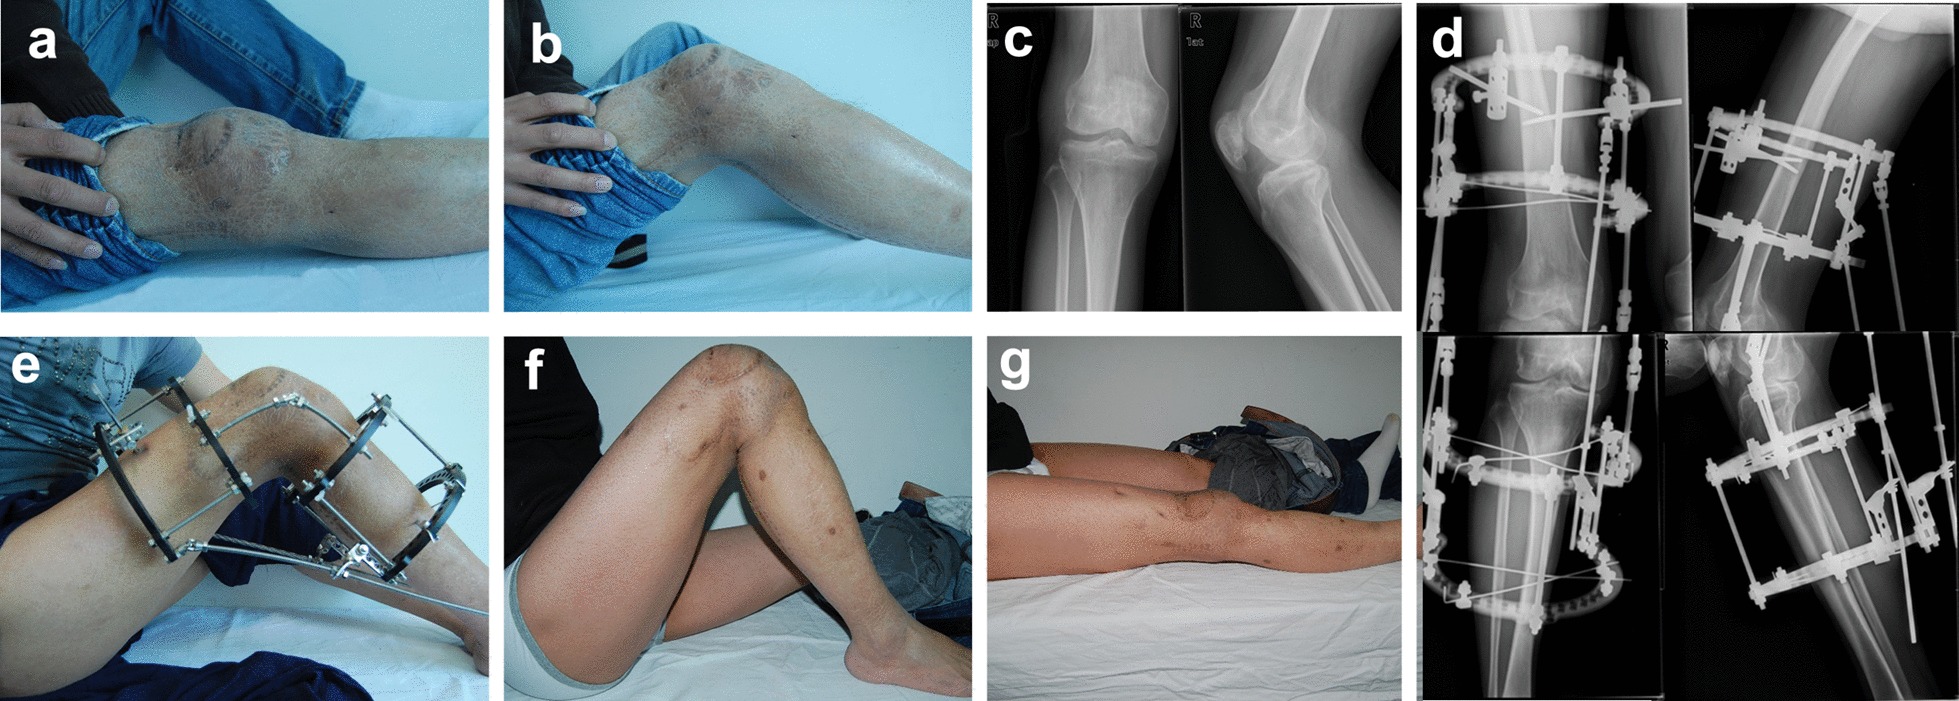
a, b Right knee ROM was 0°–40° at 1 year after first surgery; c no bone destruction observed in radiograph at follow-up; d frontal and lateral radiographs of right knee after fixation with a Ilizarov frame; e Ilizarov ring external fixation was implanted into the right tibia and femur, with the external fixation connected by hinges; f, g follow-up at 7 weeks after external fixation removal, right knee maintained ROM of 0°–130°
